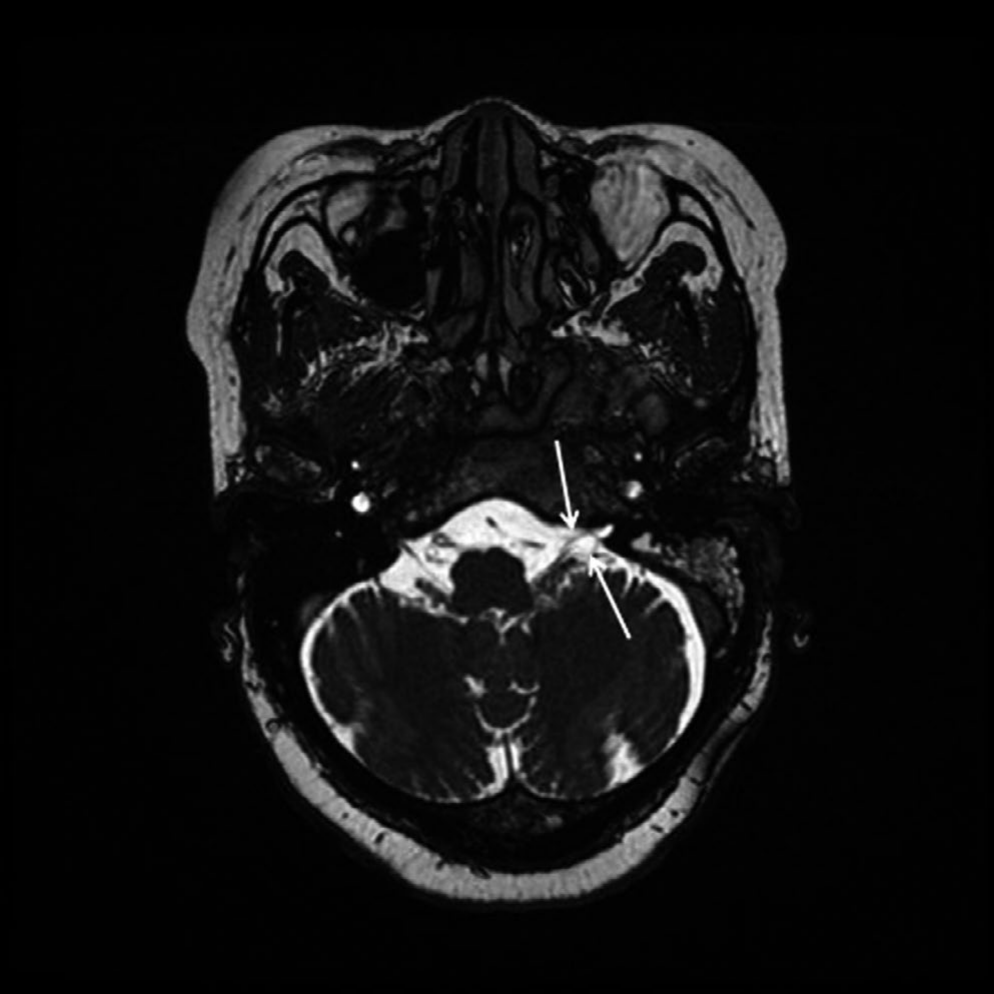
T2‐weighted thin‐slice FIESTA MRI sequence shows thickened left glossopharyngeal and vagus nerves (open arrows) entering a narrowed jugular foramen pars nervosa
